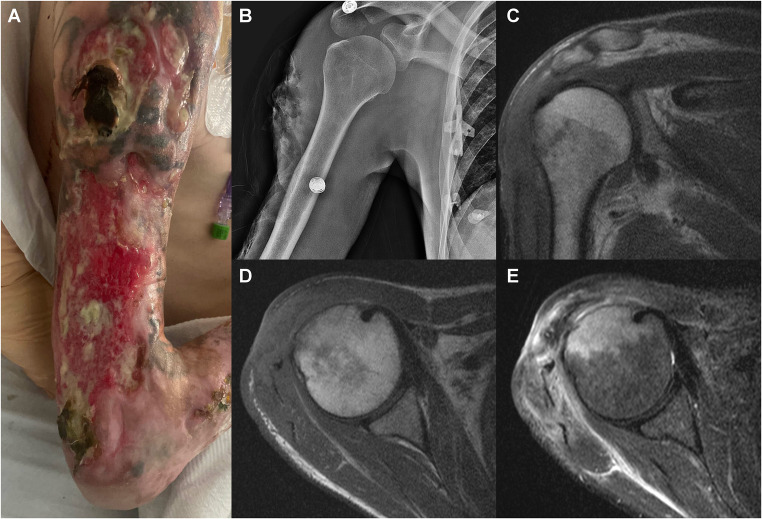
38-year-old man with an extensive xylazine-associated soft-tissue wound involving the right shoulder (A). (B) Radiograph demonstrates a soft-tissue defect and ulceration along the lateral shoulder and proximal upper arm. Coronal T1-weighted (C), axial T1-weighted (D), and axial short-τ inversion-recovery (STIR) (E) MR images demonstrate T1-hypointense marrow replacement involving the greater tuberosity, with a larger surrounding zone of marrow edema, consistent with acute osteomyelitis with adjacent reactive osteitis
